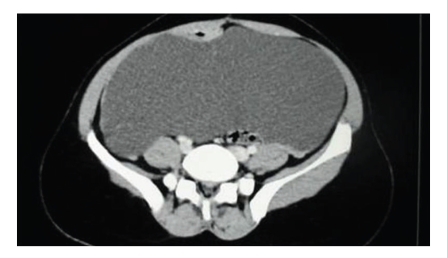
Appearance of large mesenteric cyst on abdominal CT.
